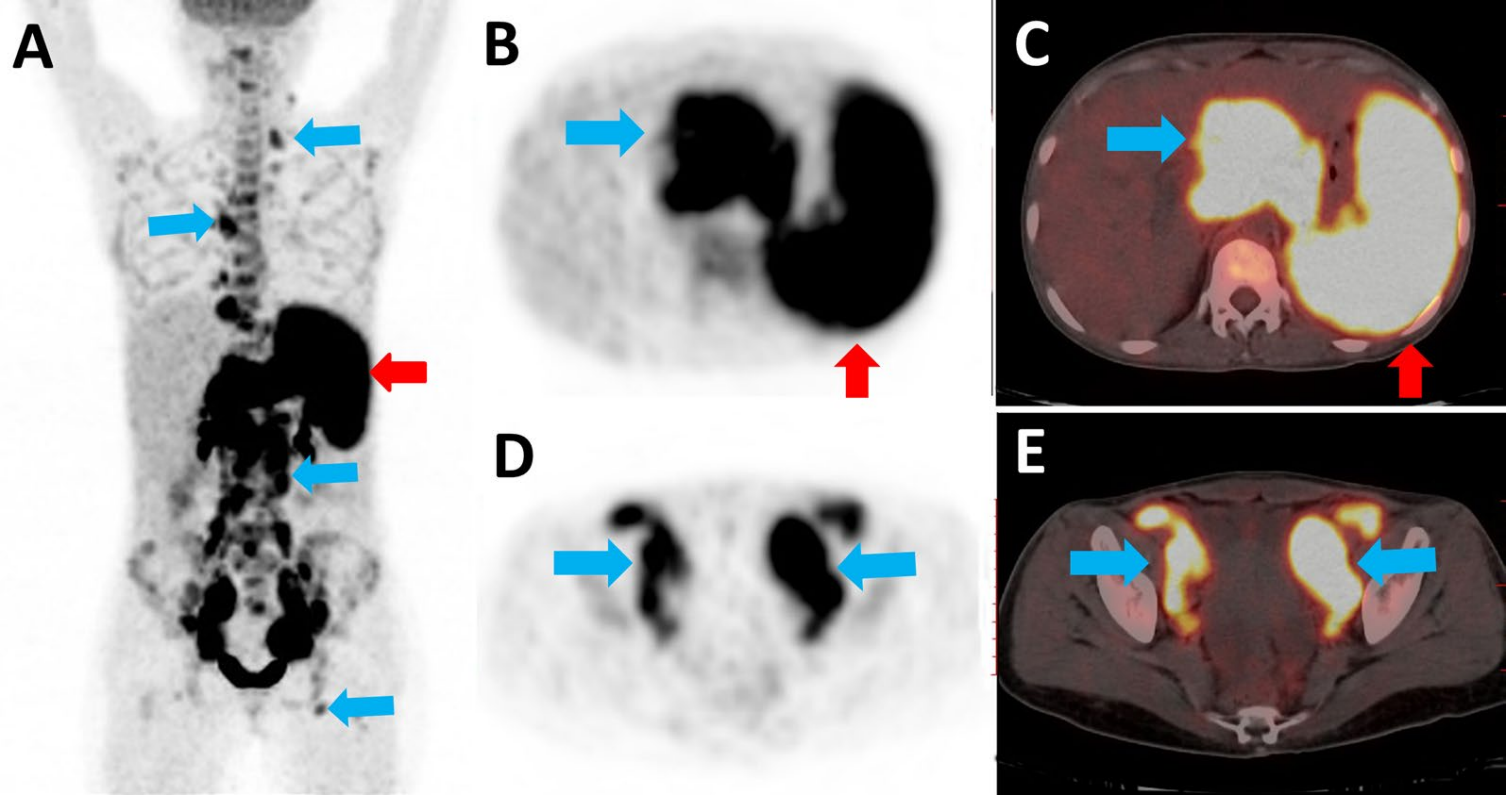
A 27-year-old female patient with a two-year history of HARRT presented with upper abdominal pain, vomiting and diarrhea. The initial ^18^F-FDG PET/CT maximum intensity projection (A PET) and axial slices (B, D PET; C, E PET/CT) showed hyper-metabolic lymph nodes in the neck, mediastinum and retro-peritoneum (blue arrows) in addition to the spleen (red arrows). The SUV_max_ of lymph nodes and spleen was 18.6 and 8.8, respectively. The neck lymph node biopsy confirmed diffuse large B-cell lymphoma
